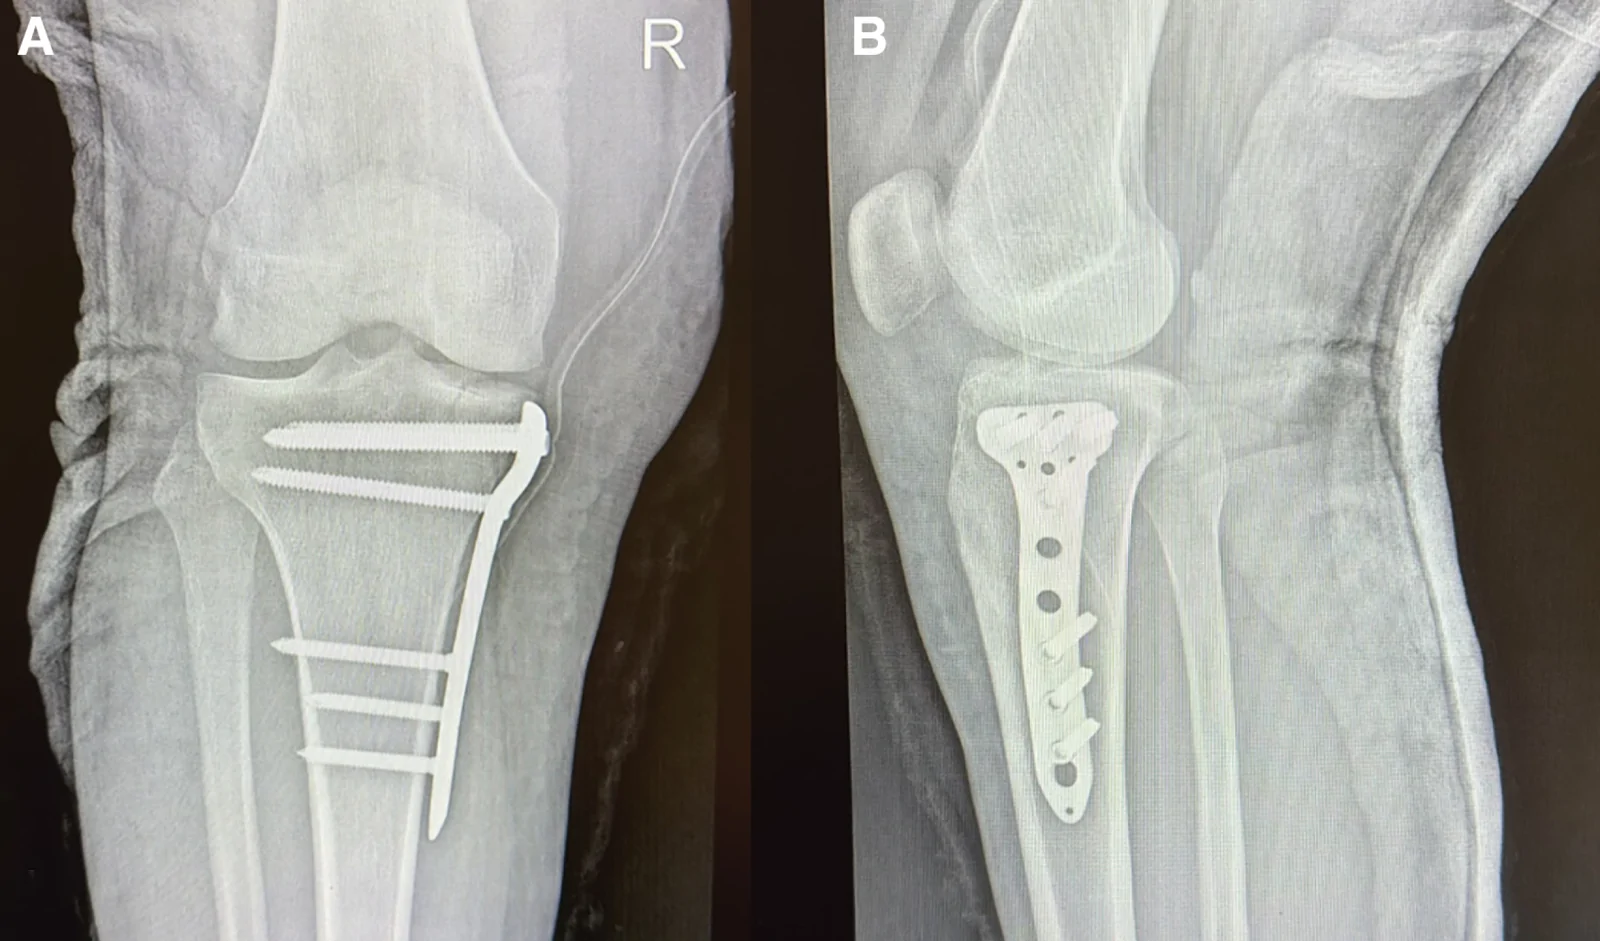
Immediate postoperative radiographs. (A) AP radiograph demonstrating anatomic reduction of the articular surface and stable fixation using a proximal medial locking plate; (B) lateral radiograph confirming restoration of the joint surface and stable subchondral support without residual displacement. AP = anteroposterior.
